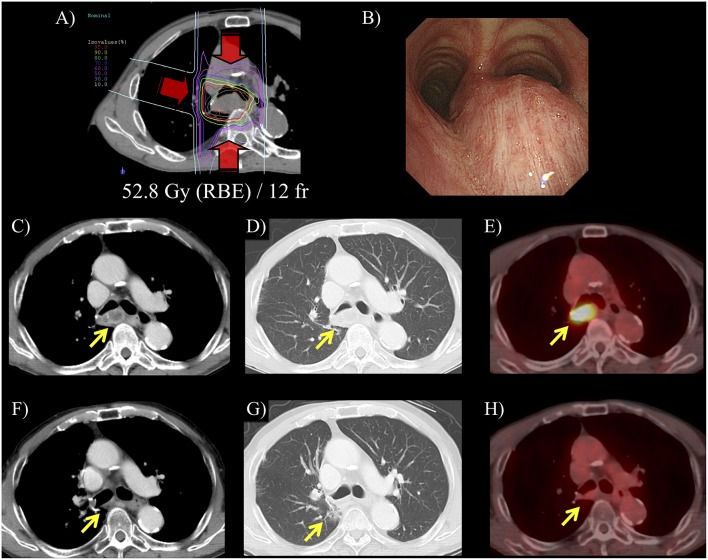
A representative case of carbon-ion radiotherapy (RT) for postoperative isolated lymph node metastasis. Patient was a 72-year-old male and mediastinal lymph node metastasis was detected at 2-year after surgery for primary lung tumor. (A) Hypofractionated carbon-ion RT was performed for the lymph node metastasis using 52.8 Gy (RBE) in 12 fractions for 3 weeks. (B) Before carbon-ion RT, bronchoscope revealed an extrinsic mass effect in the trachea. (C,D) CT showed that isolated mediastinal lymph node metastasis was 35 mm. (E) 18-fluorodeoxyglucose-positron emission tomography (FDG-PET) showed that maximum standardized uptake value was 7.6. in this lesion. (F) After carbon-ion RT, CT showed that the lymph node metastasis was diminished. (G) Pulmonary window image showed limited radiation fibrosis at the irradiated area. (H) FDG-accumulation in the lymph node was diminished and this patient had 2-years disease-free survival after the carbon-ion RT.
